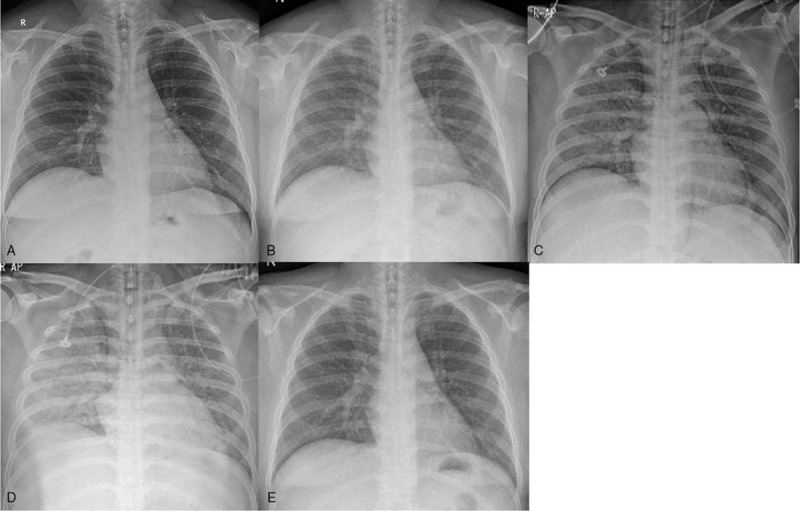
Radiological evolution of miliary tuberculosis with complicated acute respiratory distress syndrome in a 36-year-old male under corticosteroid for treatment of adult onset still's disease. (A) On the day of his visit to the emergency department, postero-anterior chest radiograph did not show any pathological findings. (B) Two weeks after initiation of corticosteroid therapy, subtle nodular opacities appeared in both lung fields on postero-anterior chest radiograph. Over the next 2 to 3 days, a rapid deterioration of clinical and radiological conditions ensued with (C) aggravation of diffuse nodular infiltrates and increased opacity in both lung fields, which (D) further progressed to diffuse alveolar involvement, showing “white lung” appearance. (E) Upon discharge, chest radiography showed complete resolution of the pathologic findings.
